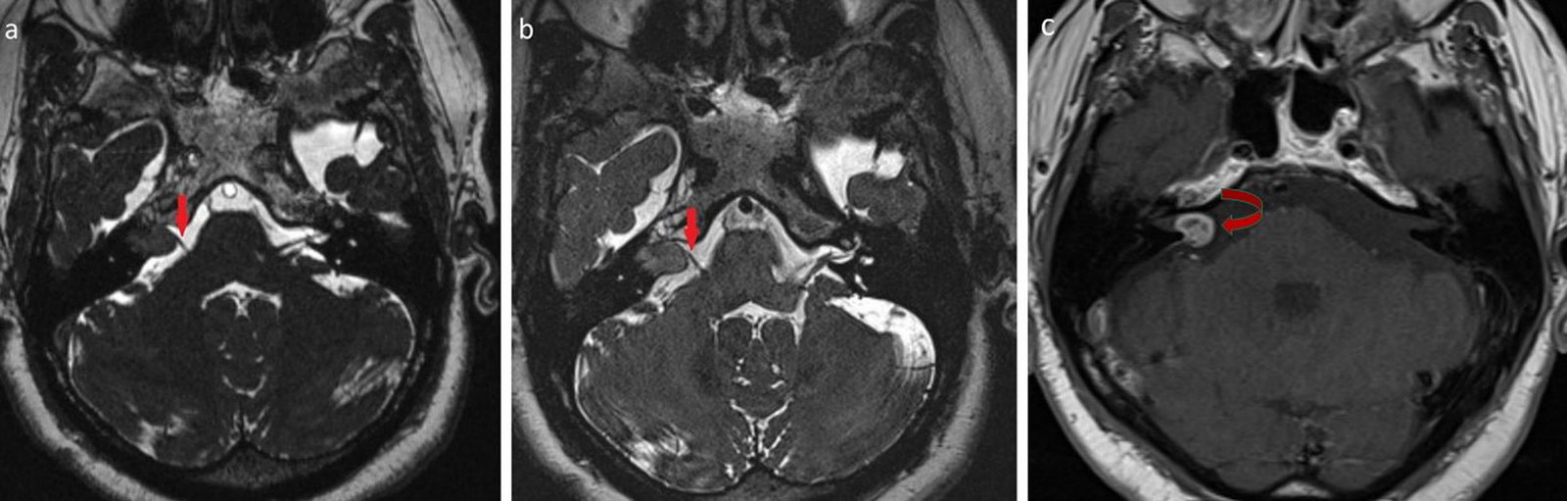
Axial T2-weighted 3D-CISS (a) and 3D-SPACE (b) images of vestibular schwannoma within the right internal auditory canal with extracanalicular extension. The lesion is clearly visualized with each sequence; however, the visibility of the relationship between the mass and cranial nerve is better on 3D-SPACE images (arrows). Axial T1-weighted postcontrast image (c) shows enhancement of the cerebellopontine angle lesion (curved arrow).
